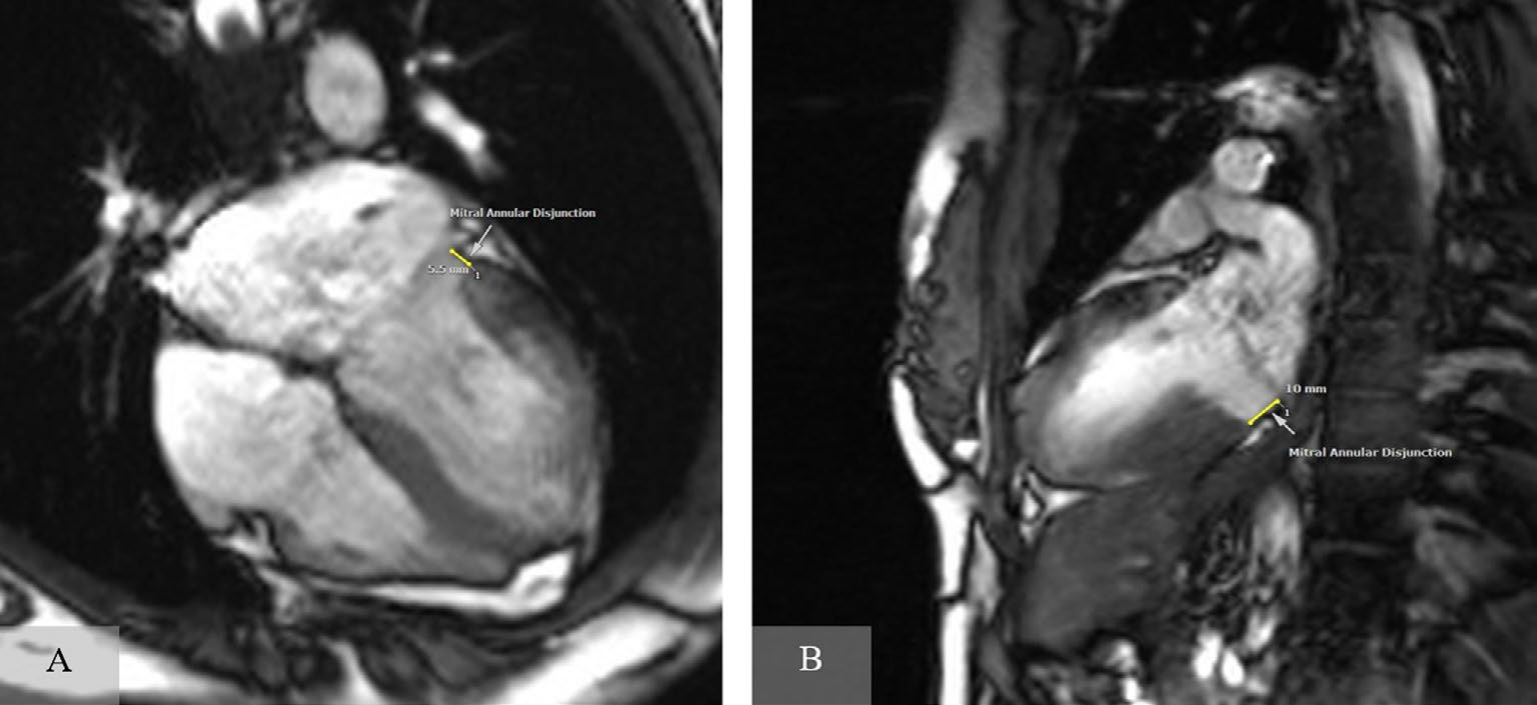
Cardiac MRI showing mitral annular disjunction, without any myocardial inflammation or scarring. (A) Four chamber view showing a 5.5 mm mitral annular disjunction. (B) Vertical long axis view showing a 10 mm mitral annular disjunction.
